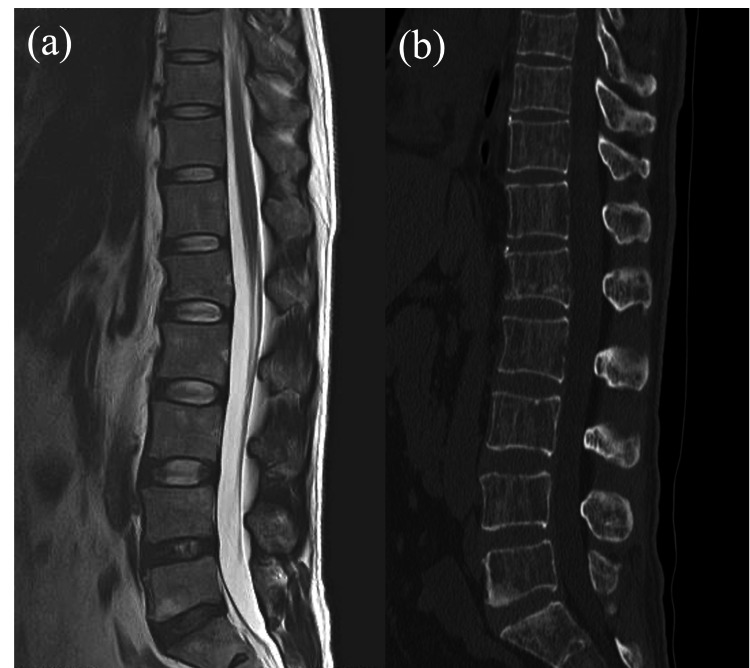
Initial MRI and CT scan. Initial MRI (a) and CT scan (b) showing no obvious L2 vertebral fracture. A slight deformation at L1 was observed on CT, with no signal change on MRI, suggesting an old fracture at L1.
